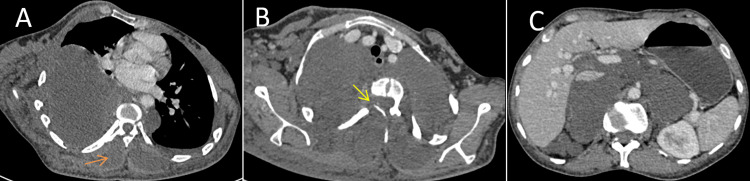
Post-contrast thoracoabdominal CT scan. CT scan, (A-C) axial sections, showing hypodense paravertebral masses, mostly necrotic with extension through the intervertebral foramen into the spinal canal next to T3 (yellow arrow), compressing lung and surrounding the abdominal aorta, associated with multiple subcutaneous and muscular lesions (orange arrow).
